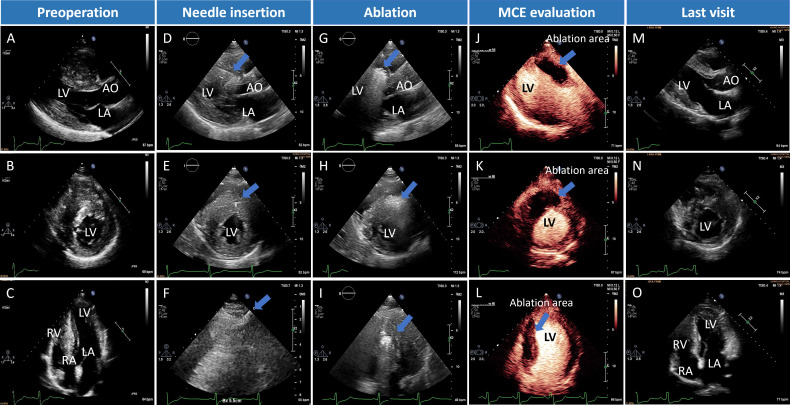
Echo imaging of percutaneous intramyocardial septal radiofrequency ablation (PIMSRA) in non-obstructive hypertrophic cardiomyopathy (NOHCM). (A–C) Preoperative echocardiography. (D–F) Intraoperative needle insertion echocardiography; arrows show the location of the radiofrequency ablation needles. (G–I) Echocardiographic images during ablation (hyperechoic zone). (J–L) Contrast echocardiography confirmed the perfusion defects in the ablated regions in the interventricular septum (IVS) immediately after the procedure. (M–O) Postoperative echocardiography. AO, aorta; LA, left atrium; LV, left ventricle; MCE, myocardial contrast echocardiography; RA, right atrium; RV, right ventricle.
